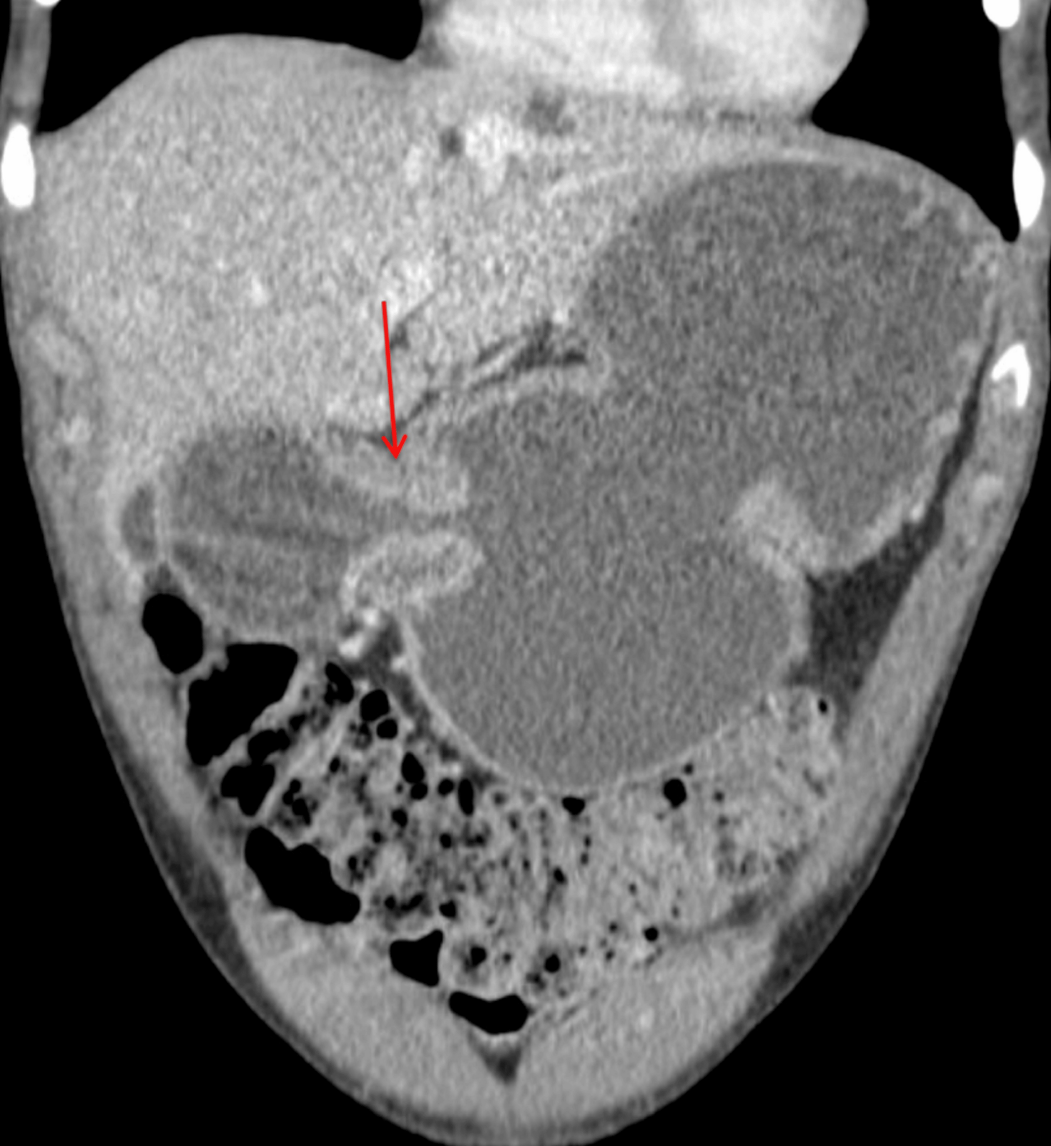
CT enterography (coronal view) with a distended stomach and stricture at the pyloric channel (red arrow) consistent with gastric outlet obstruction.
